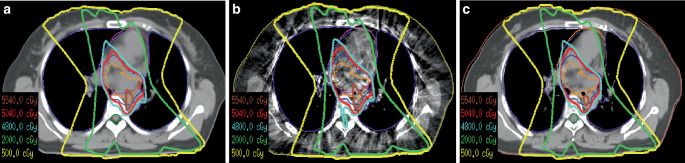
The isodose lines shown on the same transverse slice of (a) deformed CT (*dCT*), (b) cone-beam CT (*CBCT*), and (c) synthetic CT (*sCT*) calculated by the same treatment plan for one testing patient
